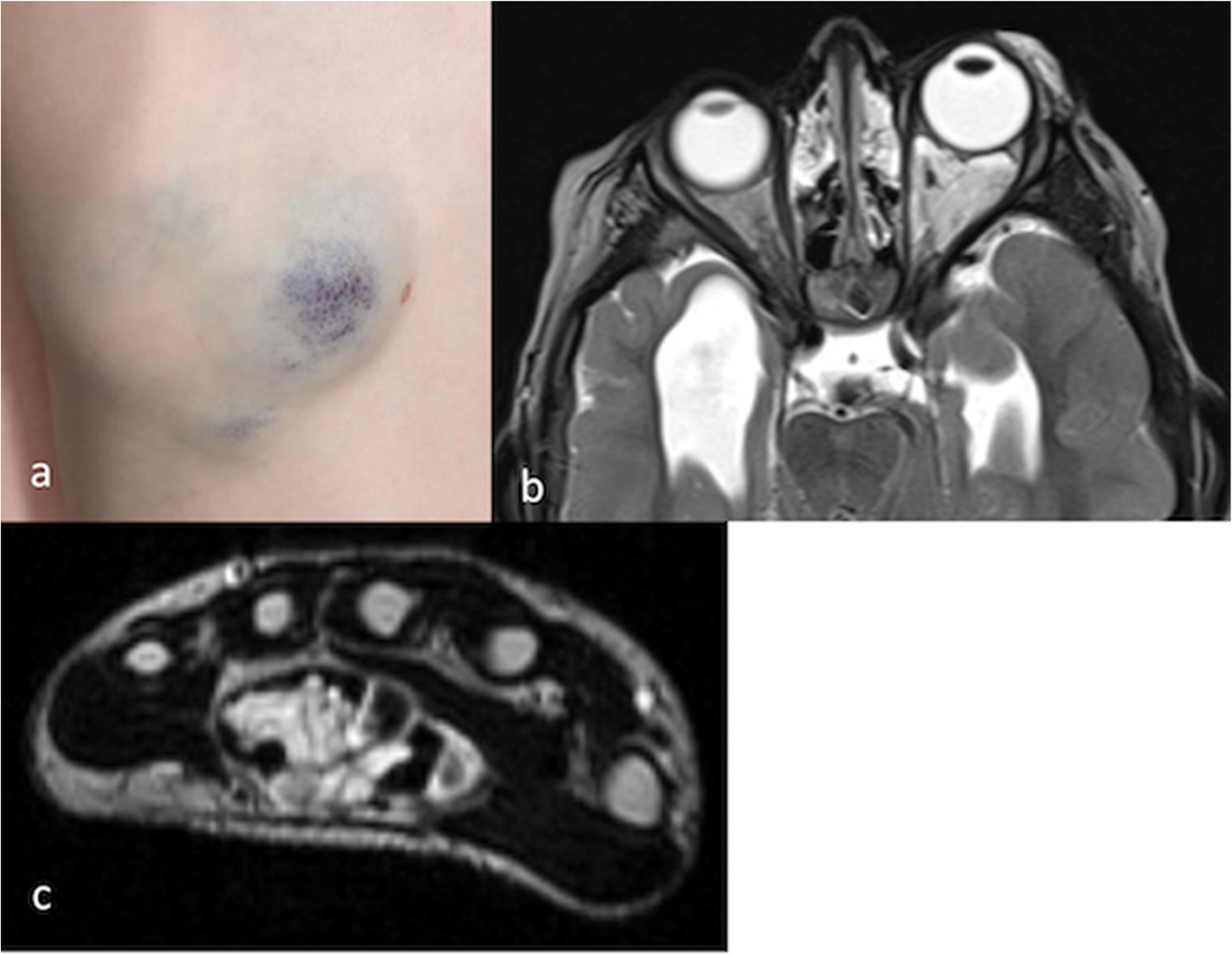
Venous malformations (VMs) that may be better suited to bleomycin sclerotherapy than with other agents a VM with a superficial component involving the dermis, which is likely to blister with other agents; b axial T2 weighted MR image of a VM of the left posterior orbit and eyelid in a 3 year old female. Intracranial abnormalities are also noted; c axial T2 weighted MR image of the wrist in a 14 year old female. The VM lies within the carpal tunnel itself
